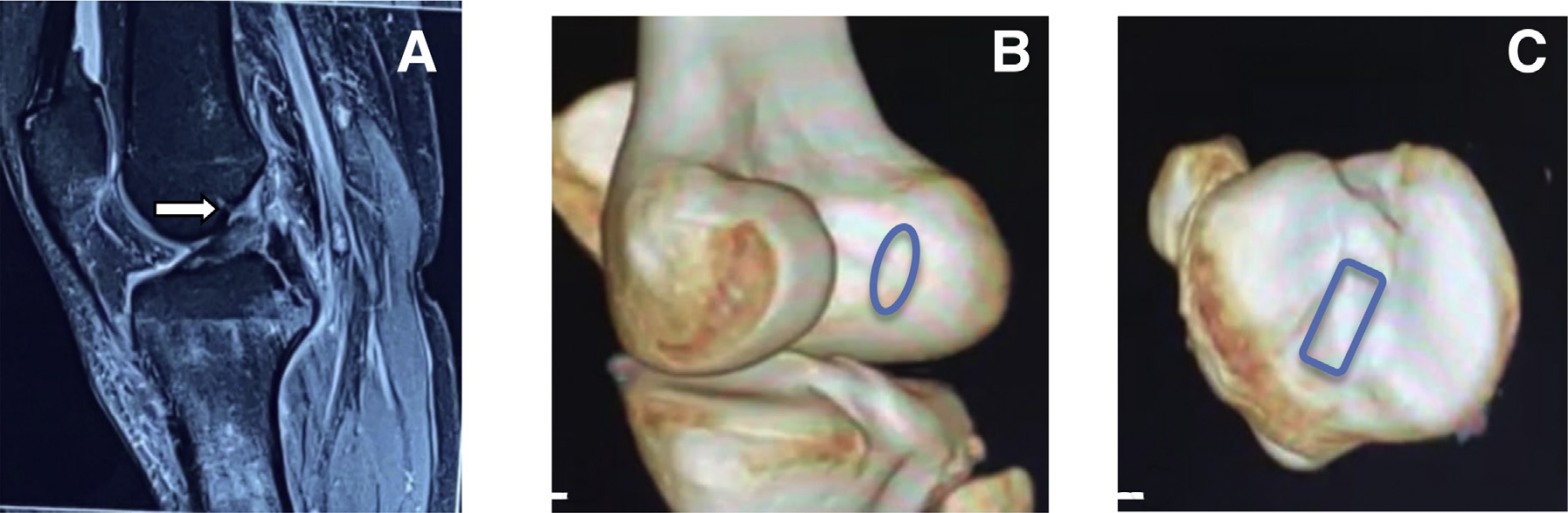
A young man with knee instability, clinically Lachman and anterior drawer grade III, suggestive of anterior cruciate ligament (ACL) injury. Preoperative MRI and 3D-CT showing complete full-thickness ACL tear from the femoral side and the footprint anatomy. (A) Sagittal PDFS MRI of right knee demonstrates ACL tear shown by a white arrow. (B) Preoperative 3D-CT scan of the right knee demonstrating an oval femoral footprint as marked by the blue oval. (C) Preoperative 3D-CT scan of the right knee demonstrating a rectangular tibial footprint as marked by the blue rectangle. PDFS, proton density fat suppressed; MRI, magnetic resonance imaging; 3D-CT, 3-dimensional computed tomography.
